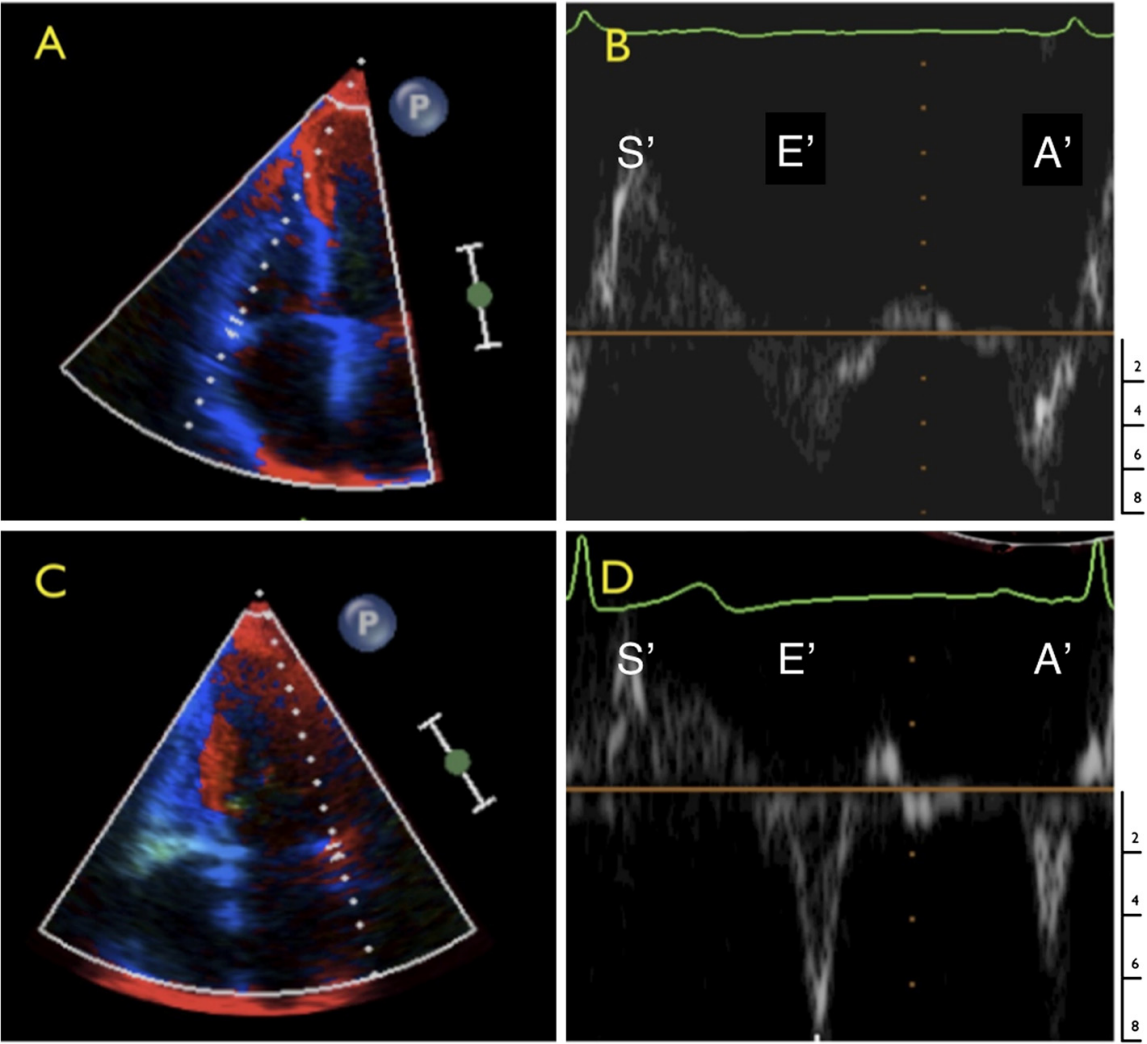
Tissue Doppler waveforms of the right (A) and left (C) atrio-ventricular grooves (Circumflex and Right coronary arteries). The right coronary artery E’ and A’ waves (B) tend to be broader leaving a shorter period of minimal cardiac motion. While the magnitude of each wave varies between individuals, the integral of the wave, or total motion is usually greater for the right than circumflex coronary arteries. This is the corollary of the rapid and more prolonged period of motion of the right coronary artery as may be seen during cine coronary angiography [7]. The duration of the PMCM of the right coronary between the E’ and A’ is also reduced relative to the Circumflex artery (D). Shown with indicative scale (cm/s). S’ indicates systolic myocardial velocity.
